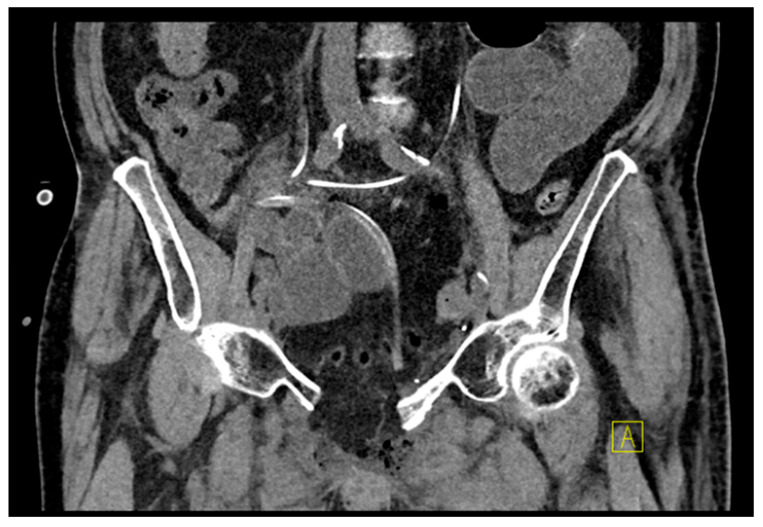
CT scan showing the resected symphyseal area with bony defect and multiple double J or ileostomy catheters after cystectomy for vesico-symphyseal fistula and chronic SAS.
